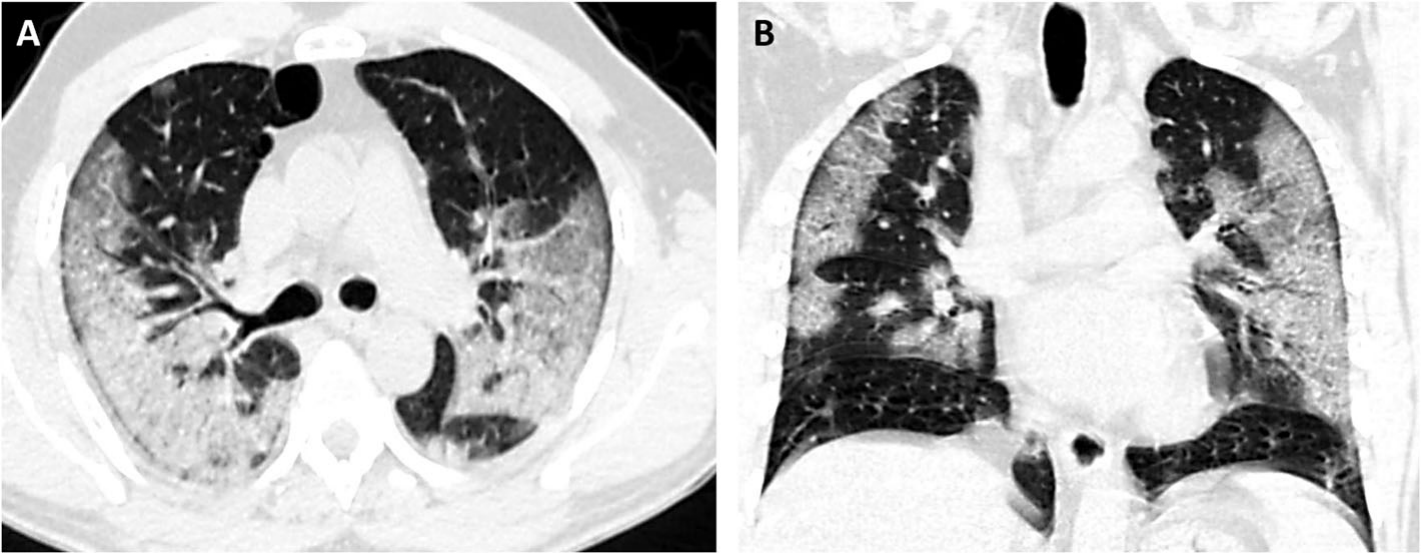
Non-contrast CT done 6 days after initial complaint of a 68-year-old male with high grade fever and tachypnea, rt RT-PCR +VE. (A, B): A, axial and B, coronal non-contrast CT scan lung window show bilateral peripheral ground glass density with thickened interlobular and intralobular lines (crazy-paving pattern) involving upper, middle lobes, and lingula
